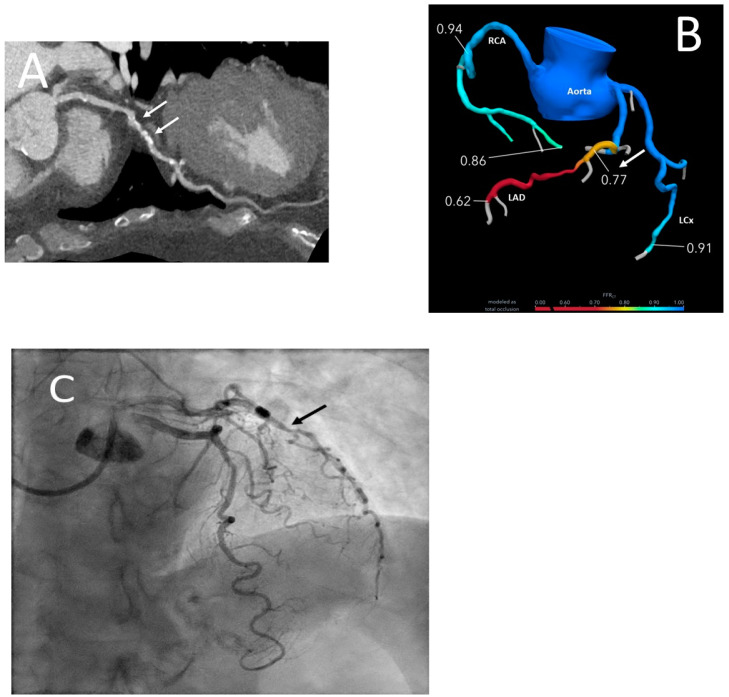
Series shows a CT coronary angiogram with curved planar reformat image (A) demonstrating moderate narrowing (arrow) of the proximal-mid left anterior descending coronary artery (LAD). Subsequent CT-fractional flow reserve (CT-FFR; B) which revealed hemodynamically significant stenosis with a value of 0.77 distal to the area of concern on CT angiogram (>0.80 is considered negative). Catheter angiogram (C) showing presence of moderate stenosis in the proximal-mid LAD (black arrow).
